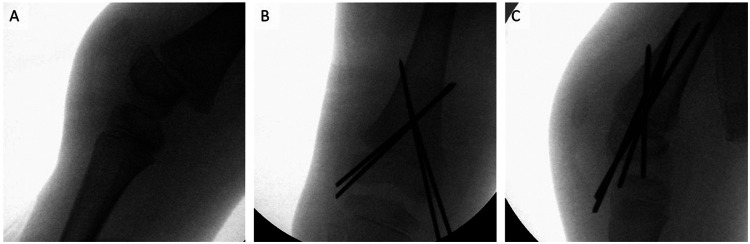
Intraoperative radiograph of the right knee with K-wires inserted. (A) Sagittal view of the intraoperative radiograph. The tibia is being held in traction. (B) Coronal view after the insertion of four 2.2-mm K-wires. (C) Sagittal view. The epiphysis is correctly aligned axially.
